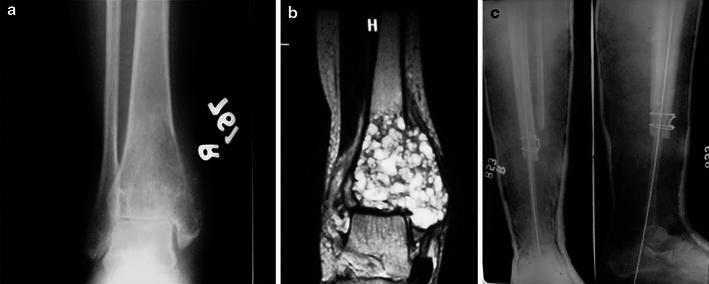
a Radiograph showing giant cell tumour in a 30-year-old female, b MRI of the same patient, c post-operative radiographs
